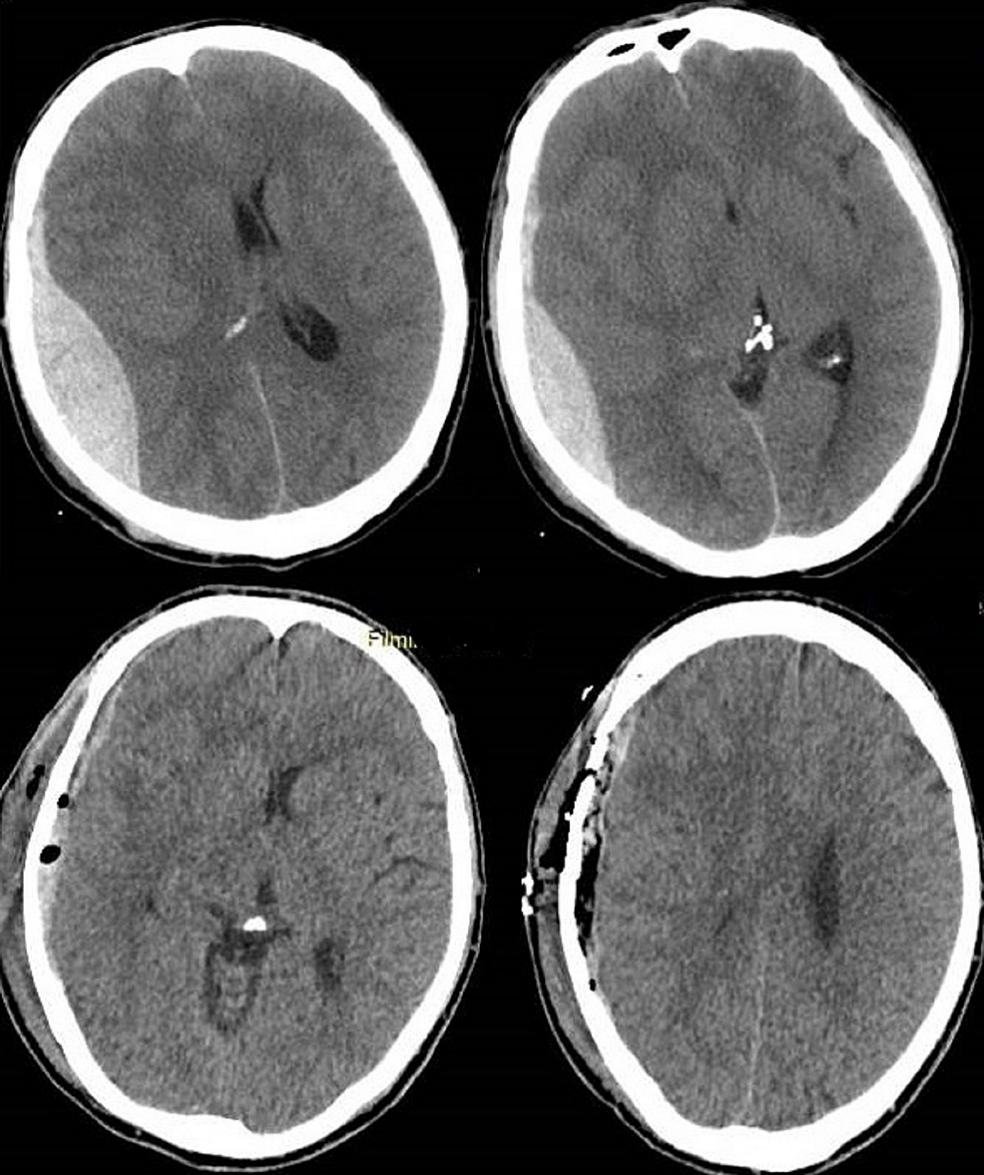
CT scan Acute epidural hematoma before and after evacuation
